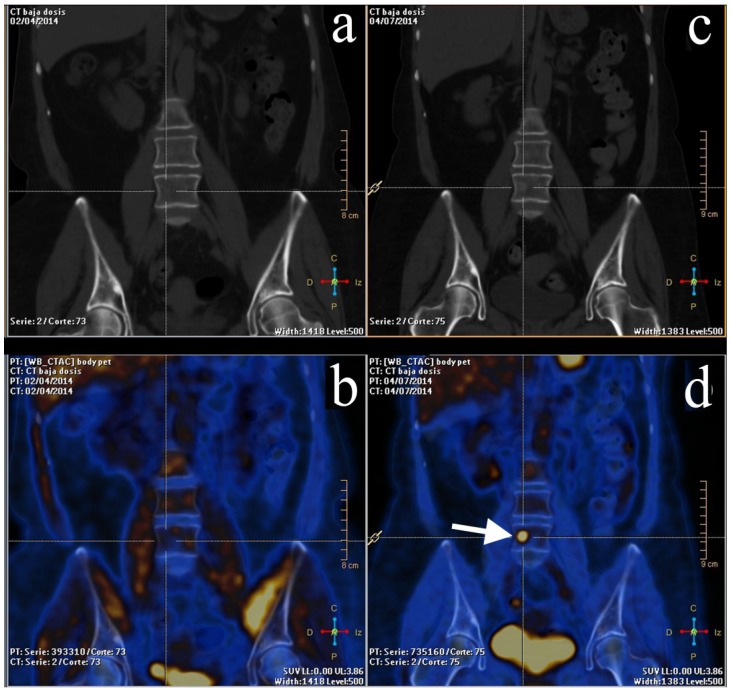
Same patient as Figure 2. *Left column*: baseline PET/CT scan. (a,b) No abnormality was noticed on CT or PET/CT. *Right column*: mid-therapy PET/CT scan. (c) Non-enhanced CT showing an iso-hyperdense rounded lesion in the fourth lumbar vertebrae, called to be as undetermined. (d) PET/CT demonstrating a focus of FDG uptake (arrow) in the lumbar vertebrae due to bone metastases.
